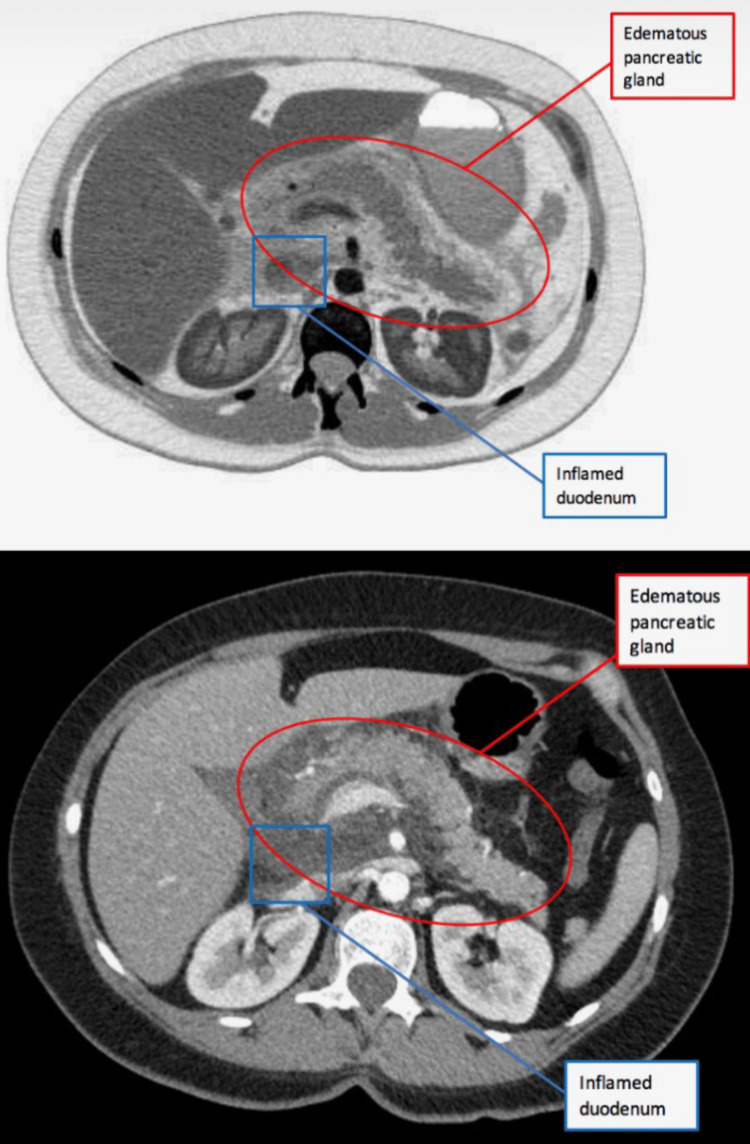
CT Scan of Abdomen in the transverse plane showed findings consistent with uncomplicated pancreatitis and inflammatory changes in the duodenum most likely as a secondary reaction to the adjacent acute pancreatitis
